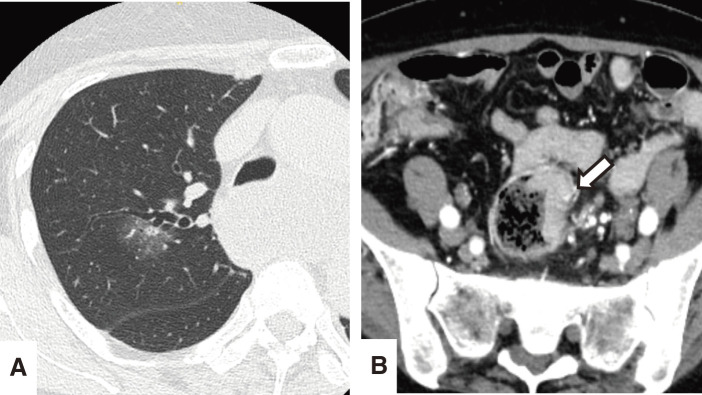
(A) A chest CT showing a 26-mm part-solid nodule in the right upper lobe. (B) An abdominal CT showing an irregular wall thickening in the sigmoid colon (arrowhead), which was later diagnosed as advanced colon cancer.
